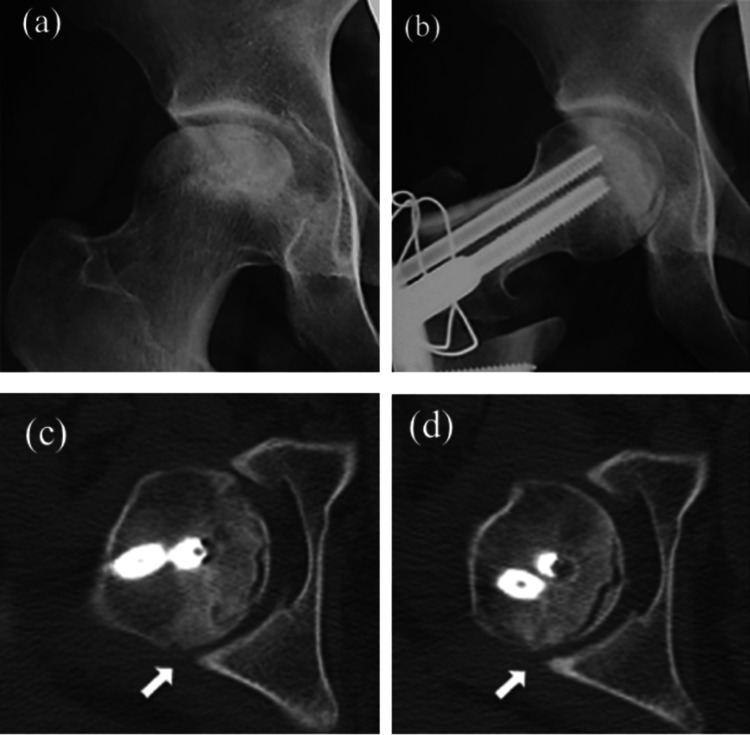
Diagnostic images and CT images of a 44-year-old male. (a) Diagnostic images of a 44-year-old male with a history of sudden hearing loss treated with high-dose corticosteroids. The pre-operative anteroposterior radiograph of the right hip shows a severely collapsed lesion and no viable area of the loaded portion. (b) A 110° posterior rotational osteotomy with 20° varization was performed. (c) CT images were acquired six weeks post-operatively. The distance from the posterior acetabular joint surface to the posterior joint surface of the femoral head in the neutral position is 3.52 mm (white arrow) on the horizontal CT image. (d) The distance from the posterior acetabular joint surface to the posterior joint surface of the femoral head in the 45° flexion position is 3.79 mm (white arrow) on the horizontal CT image. The translation of the femoral head between the neutral and 45° flexion positions is 0.27 mm, which is considered stable. CT: Computed tomography
